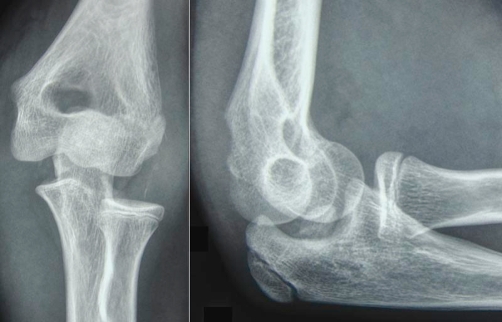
Radiographs performed after closed reduction. (A) Anteroposterior view showing incongruity of the elbow joint. (B) Lateral view. A bone fragment is clearly identified into the joint.
